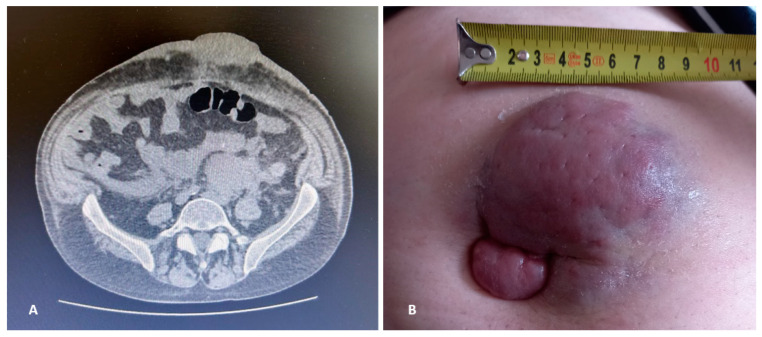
Radiological and clinical images. CT scan of the abdomen: tissue elevation of about 43 × 28 mm at the peri-umbilical site involving the soft tissues of the left anterior abdominal wall and extended to the para-umbilical region, characterized by pseudo-nodular morphology (A). Umbilical and para-umbilical lesion protruding from the umbilical scar: 80 × 60 mm, raised about 2 cm, purplish colored and with hard-rubbery consistency (B).
